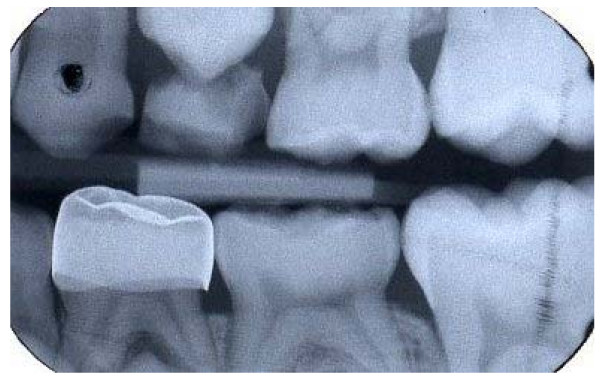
Radiograph of Hall PMC on tooth 74 (LLD) recorded as satisfactory fit. Patient randomisation number 7.
